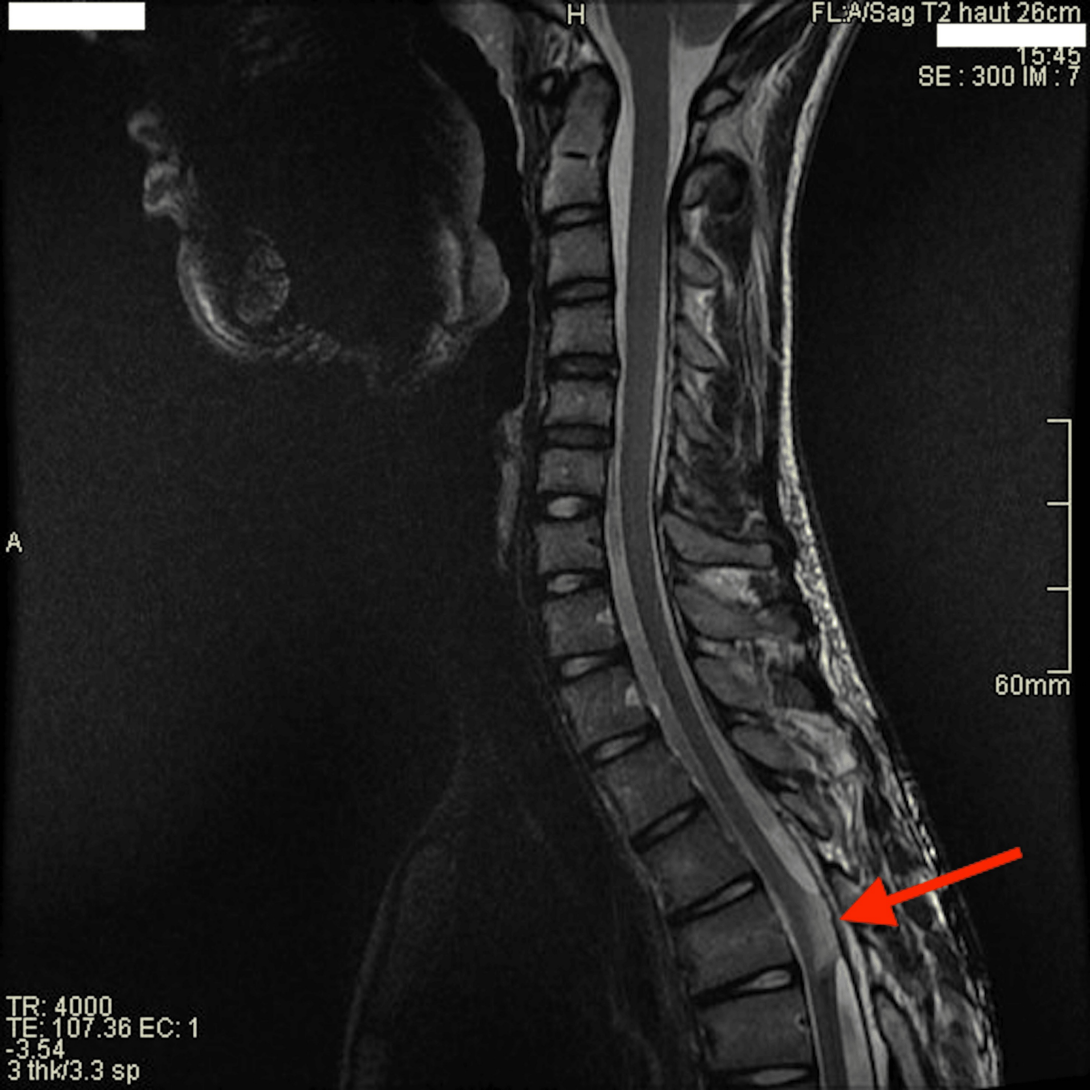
Sagittal T2-weighted image at five-year follow-up. After-effects of cavernous resection with retraction of the spinal cord backward (red arrow) at the laminotomy site.
